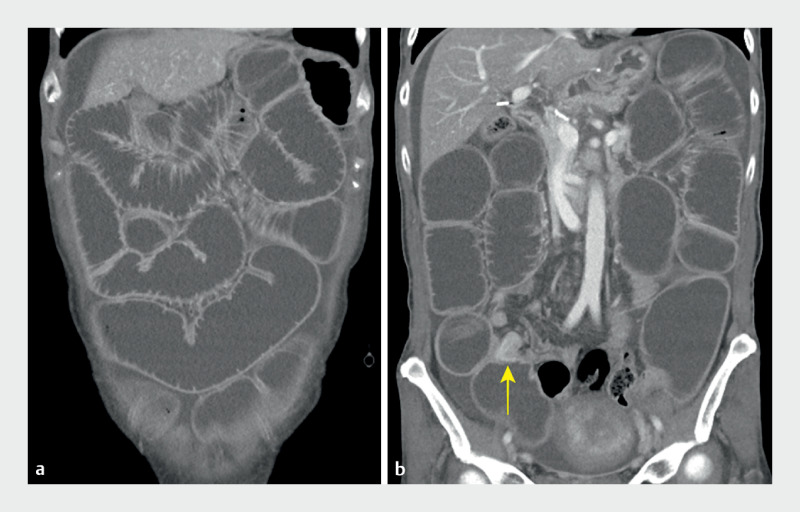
Images from the initial computed tomography scan showing:
